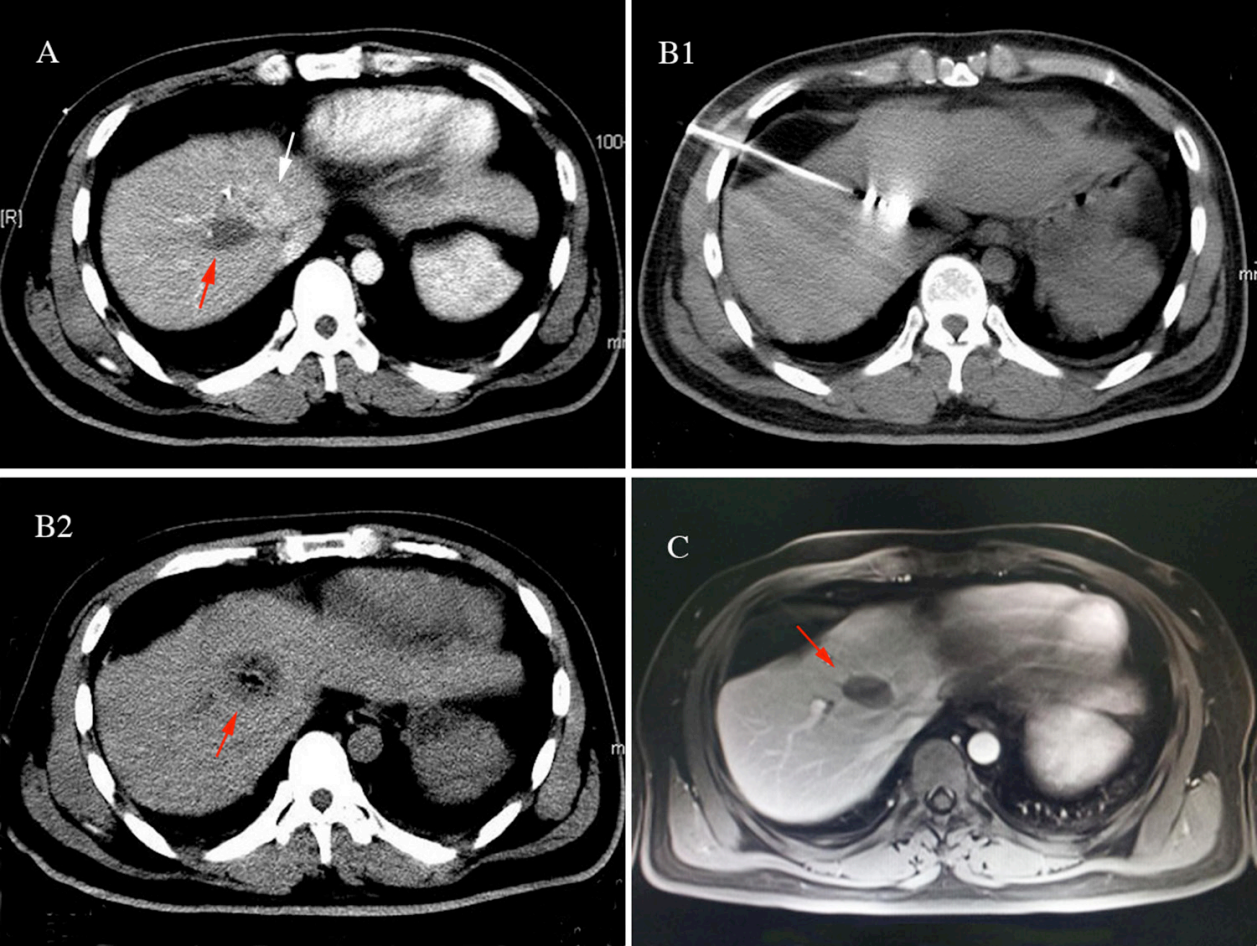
A 57-year-old female found a liver S8 metastatic tumor with diameter of 1.8cm 2 years after modified radical mastectomy of the left-side infiltrating ductal carcinoma received the ultrasound guided MWA The postoperative review implied only a small portion of lesion was ablated, the patients experienced the partially off-target effect. The patients then underwent CT-guided microwave ablation (MWA) in our hospital. There was also no indication of tumor residue or recurrence in the follow-ups. (A) Partially off-target phenomenon occurred after ultrasound-guided MWA: The contrast-enhanced CT review: a small portion of the liver S8 lesion was ablated (white arrow), but there was a low-density area (red arrow) beside the lesion. (B) CT-guided MWA for liver tumor near the diaphragm: B1: MWA antenna precisely inserted into the tumor during the ablation process, with its needle tip slightly exceeded the edge of the tumor. B2: Contrast-enhanced CT immediately after MWA: ablation area completely covered the tumor (red arrow), without tumor residue. (C) The upper abdomen MRI review 2 months postoperatively: tumor achieved complete response without residue (red arrow).
